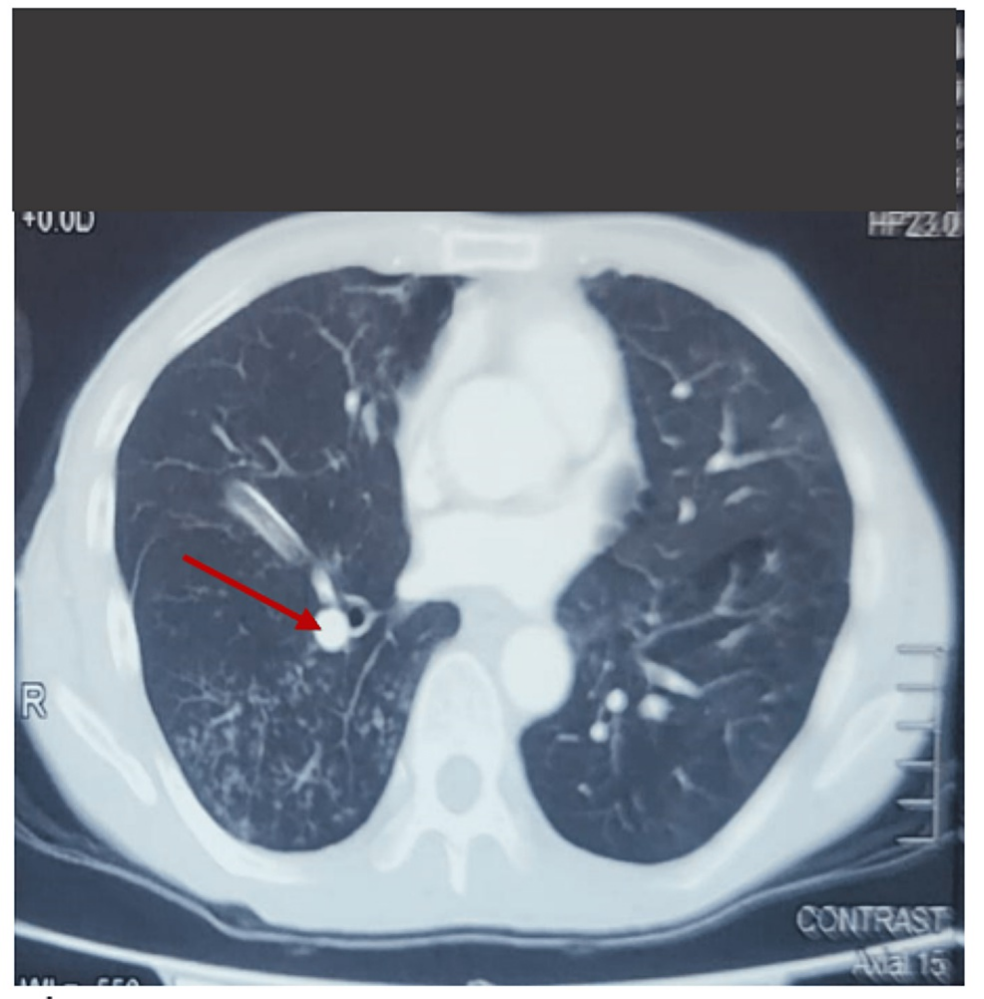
Contrast-enhanced computed tomography axial section showed a hyperdense lesion measuring approximately 1.3×1.4×1.9 cm (AP×W×CC) within the right bronchus with chronic fibrosis and cystic lung changes.
